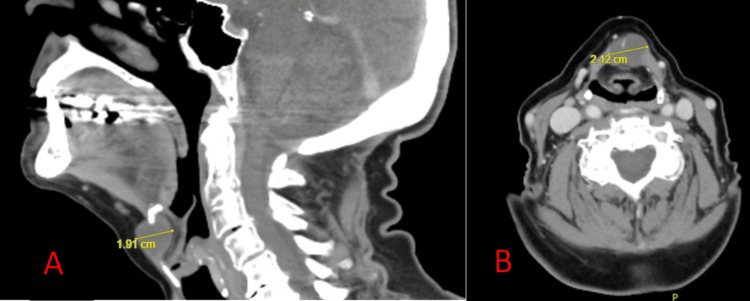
Computed Tomography (CT) Scan for Patient A Sagittal (A) and Axial (B) CT scan views for Patient A demonstrating a 1.9 cm x 1.9 cm x 2.1 cm lesion with septal calcifications embedded in the midline strap muscles with mild mass effect
